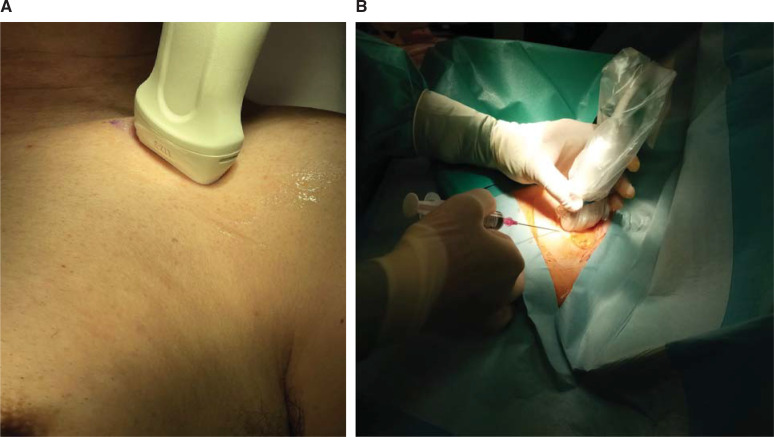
(A) Transducer, operator, and (B) needle configuration for ultrasound-guided axillary vein access in a draped representative patient. Cannulation was attempted with the ultrasound probe aligned perpendicular to the longitudinal axis of the axillary artery and vein.
